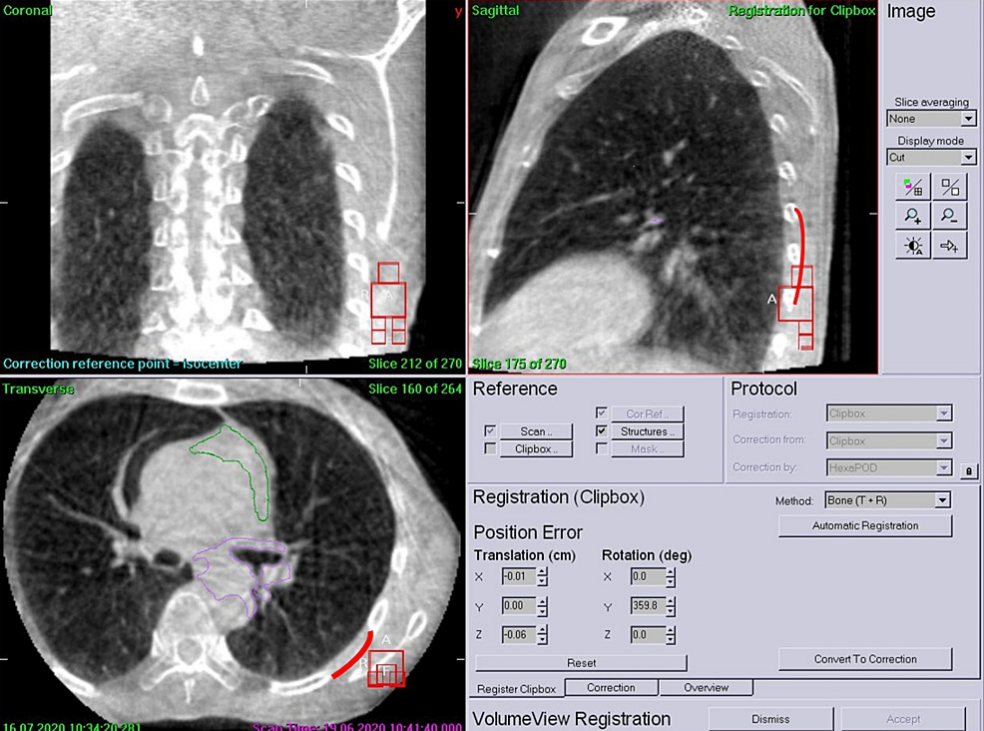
CBCT images displayed in three orthogonal planes At a cumulative dose of 43.2 Gy, CBCT suggested GGO on a sagittal plane posteriorly, as shown by red lines. Purple and green lines on the axial plane show the CTV contours of the primary tumors and the elective lymph nodes, respectively.
